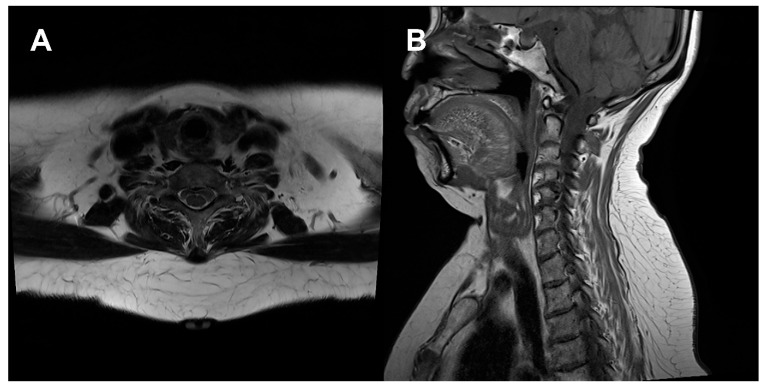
Preoperative T1-weighted magnetic resonance imaging (MRI) of patient 1. (A) Axial view demonstrating a dorsocervical fat pad with poorly defined margins relative to the surrounding adipose tissue, without a discrete encapsulated mass. (B) Sagittal view showing indistinct boundaries between the superficial and deep adipose layers, with multiple internal linear low-signal strands within the deep portion, consistent with dense fibrous septation.
